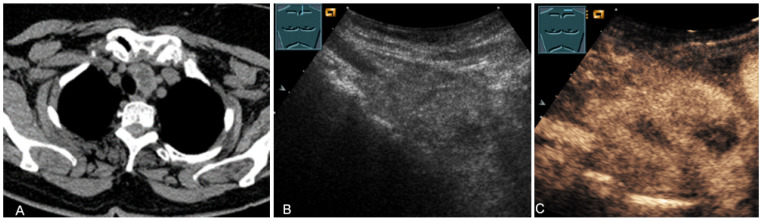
An echogenic mediastinal mass as an incidental finding on CT (provided by Prof. Dr. Andreas H. Mahnken, Marburg, Germany) (A), B-mode US (B), and CEUS (C), evaluated by imaging as a retrosternal located thyroid gland.
